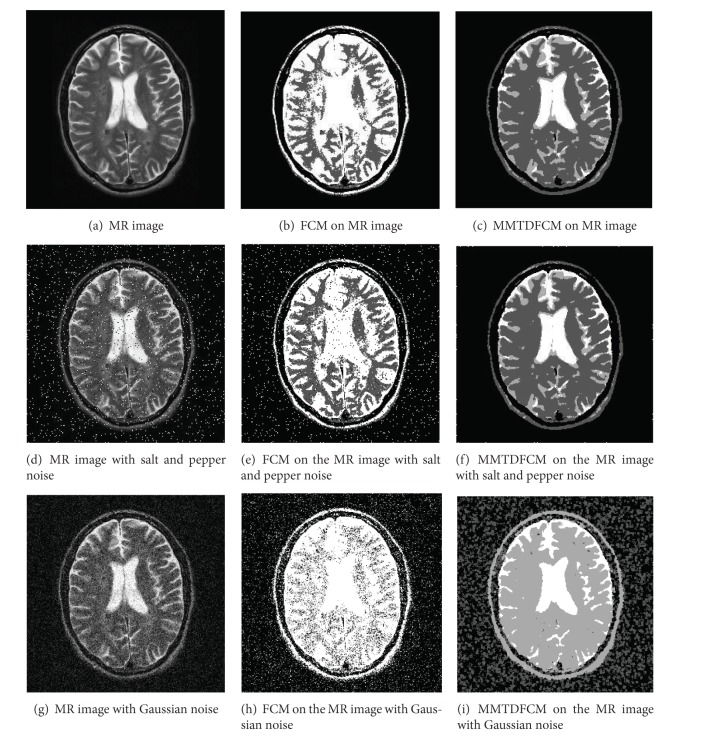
Segmentation of an MR image (*c* = 4).
